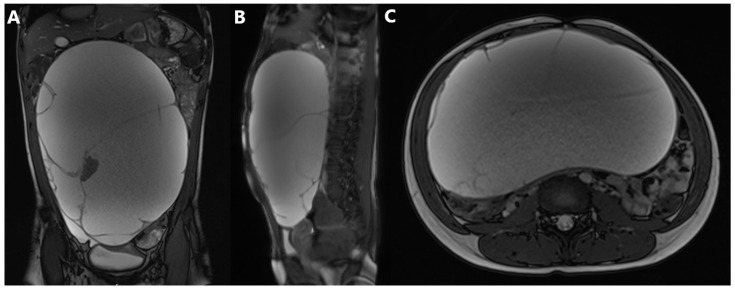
MRI of the abdomen in (A) coronal, (B) sagittal, and (C) transverse planes showing a tumor mass measuring 22cm × 13cm × 30 cm (LL × AP × CC).
